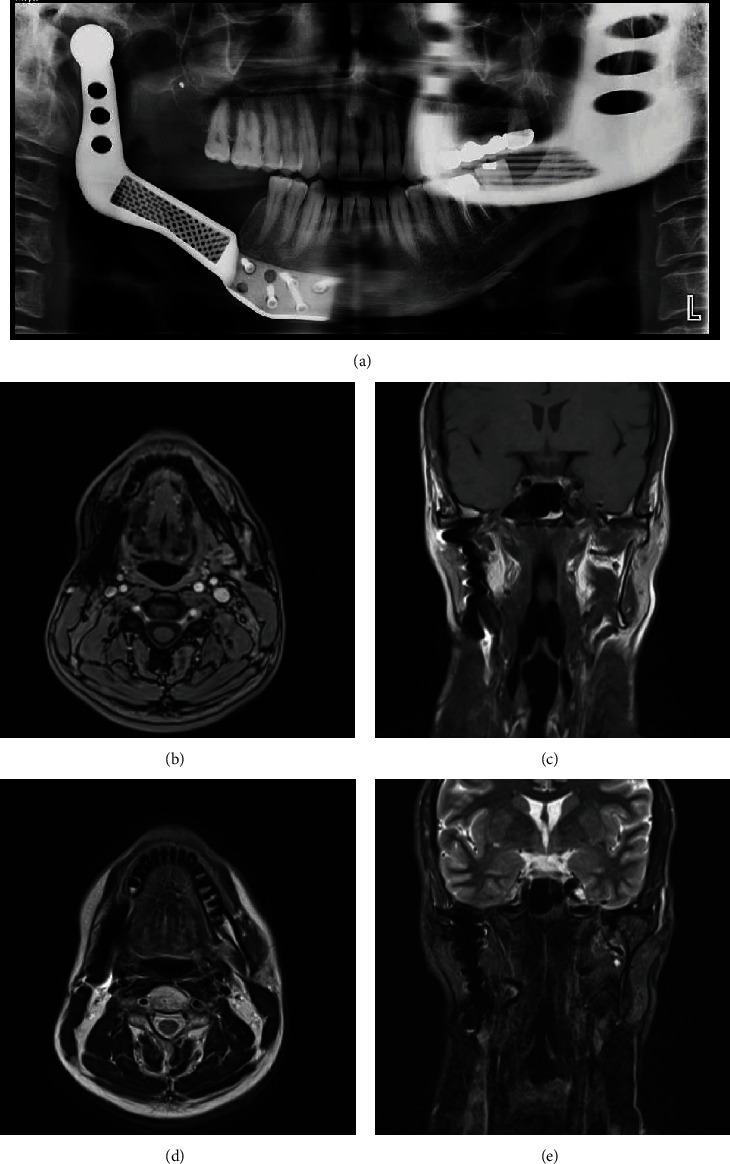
(a) Panoramic view 2 years after tumor removal. Evidence of cortication and healing is noted in the alveolar bone in the surgical bed. (b) MR images 2 years after treatment. (b, c) T1 postcontrast axial and coronal views. (d, e) T2 axial and coronal views. Evidence of previous surgery and blooming artifacts due to the prosthesis in the anatomic site of the right mandible is seen. As could be evaluated in the surgery bed, no abnormal enhancing lesion or any sign of recurrence is visualized.
